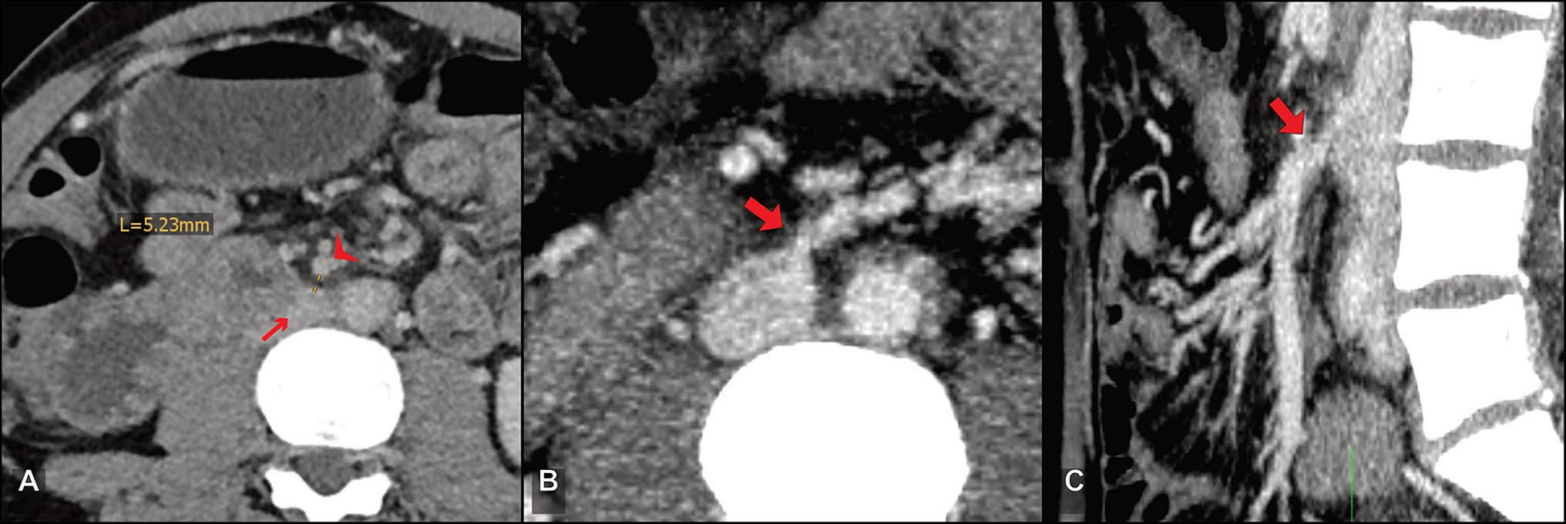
A 49-year-old male PHT patient with liver cirrhosis, extrahepatic portal vein thrombosis, and advanced symptoms—PSSS procedure: mesocaval; a preoperative CT, b postoperative CT, axial MIP reconstruction and c postoperative CT, sagittal MIP reconstruction. Small arrow: IVC; arrowhead: SMV; bold arrow: mesocaval anastomosis
